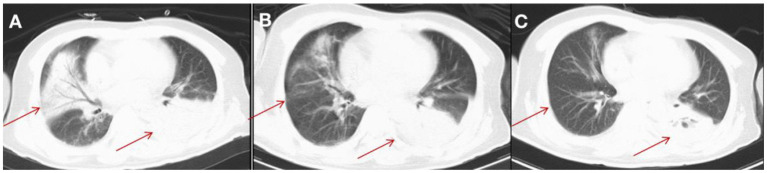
CT imaging for the patient's lung. (A) On day 25, a CT scan of the lung showed multiple inflammatory lesions (red arrow) and small amount of bilateral pleural effusion in both lungs. (B) After 2 weeks of treatment, a CT scan showed that the lesions were absorbed in the right side of patient's lung. (C) After 3 weeks of treatment, a CT scan showed that lesions were significantly absorbed in the right side of patient's lung (red arrow) and smaller amount of bilateral pleural effusion in the side of patient's lung than that after 2 weeks of treatment.
